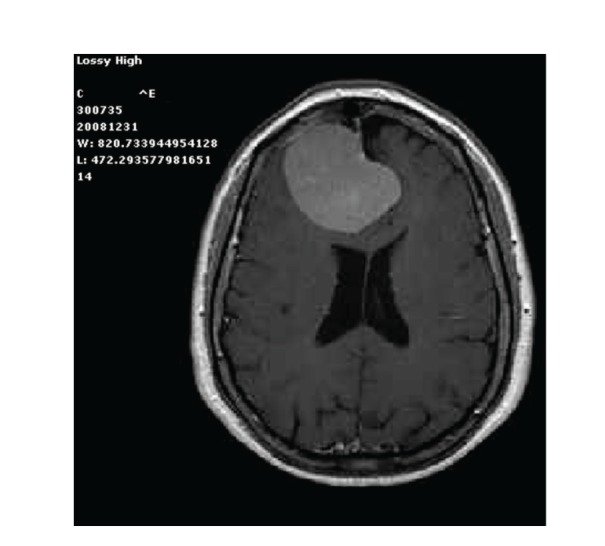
Preoperative contrast-enhanced axial MRI of the right frontal falx meningioma.
